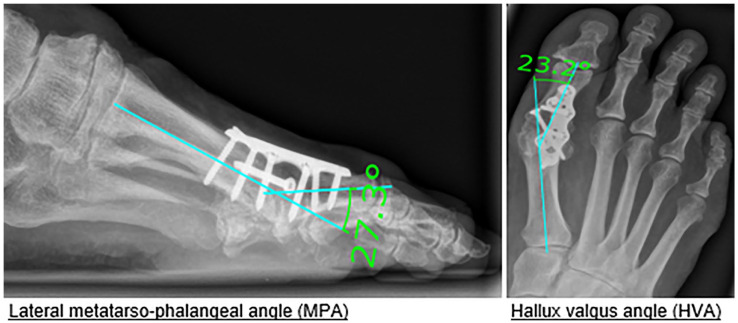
Radiographic assessment.
